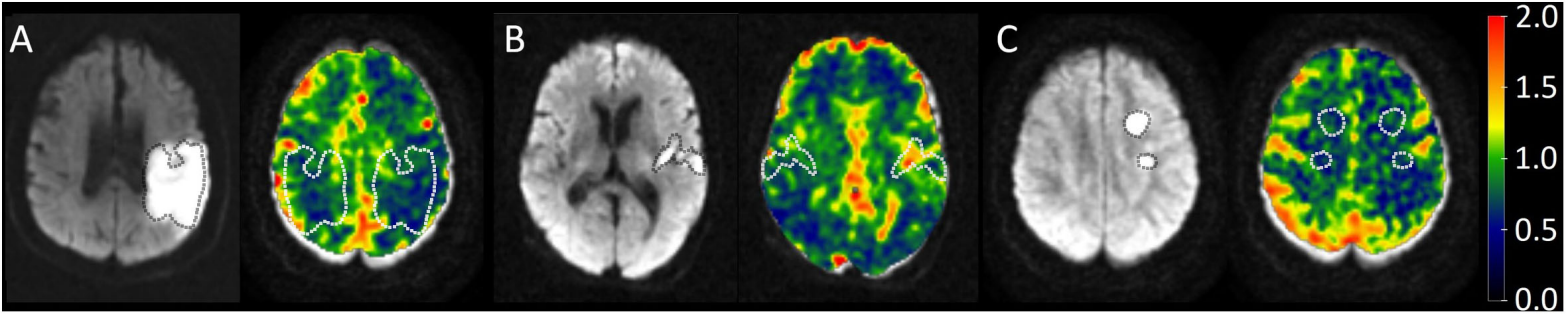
The diffusion-weighted images (DWI) on the left of each panel serve as a guide to identify the ischemic region, which is delineated with a dotted line. On the CVR maps, both the ischemic and contralateral regions are outlined to allow comparison. The color bar indicates lower CVR values in blue and higher values in red. (A) Patient 23 demonstrated decreased CVR. (B) Patient 11 showed increased CVR. (C) Patient 2 showed no significant differences in ROI CVR. Cerebro reactivity maps in three different subjects.
